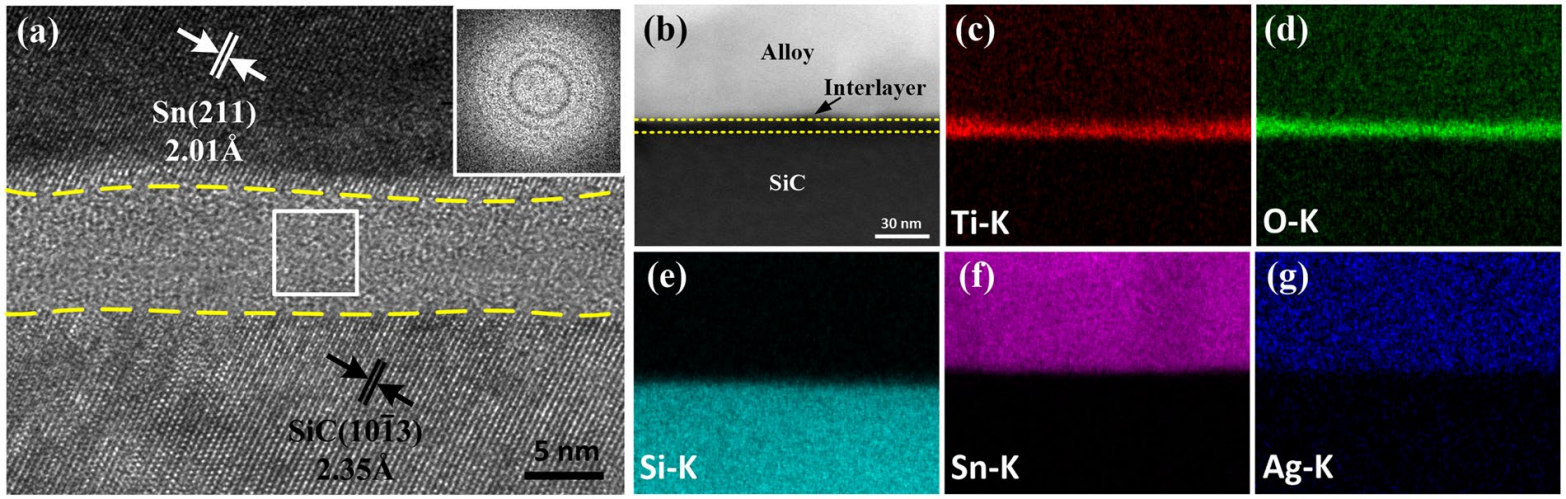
(a) High-resolution TEM image of the interface soldered with ultrasonic action for 1 s, and the inset was obtained by FFT of the white rectangular area; (b) HAADF image of the interface and the corresponding STEM-EDS maps of (c) Ti, (d) O, (e) Si, (f) Sn, and (g) Ag.
